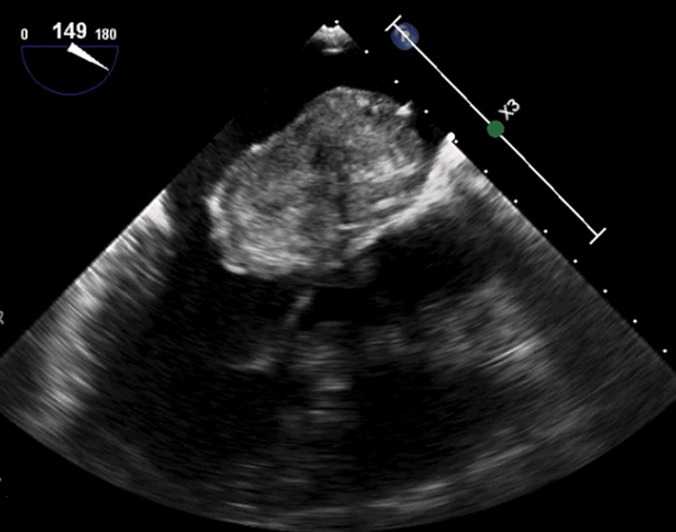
left atrial mass on 2D transoesophageal echocardiography
